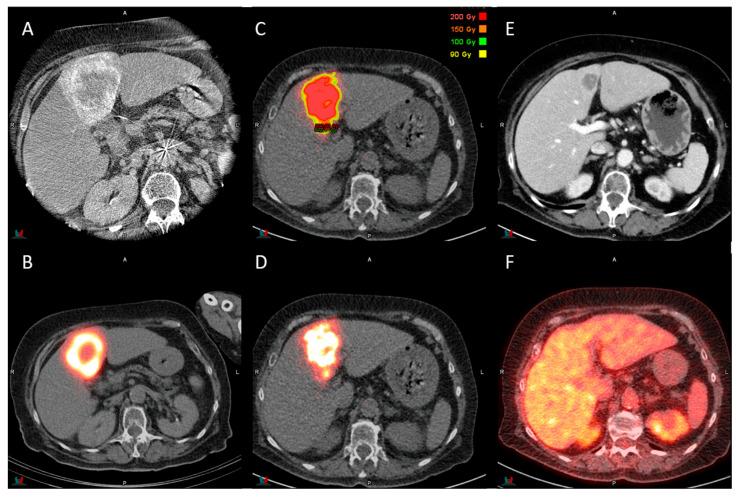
(A) Contrast-enhanced cone-beam CT of the treatment volume (segment 4) with one large colorectal metastasis before TARE; (B) ^99m^Tc-MAA SPECT/CT used for planning dosimetry showing good ^99m^Tc-MAA uptake in segment 4; (C) ^90^Y PET/CT dose maps showing a uniform dose distribution of 200 Gy in the center and a lower dose in the border of the treated volume; (D) ^90^Y PET/CT showing good tumor uptake in segment 4 (activity 1.1 GBq; planTD_mean_ 200 Gy; TD_mean_ 259.2 Gy); (E) post-treatment contrast-enhanced CT at 3 months showing a remaining lesion (partial response); (F) ^18^F-FDG PET/CT at 3 months showing no ^18^FDG uptake in the treated lesion in segment 4.
